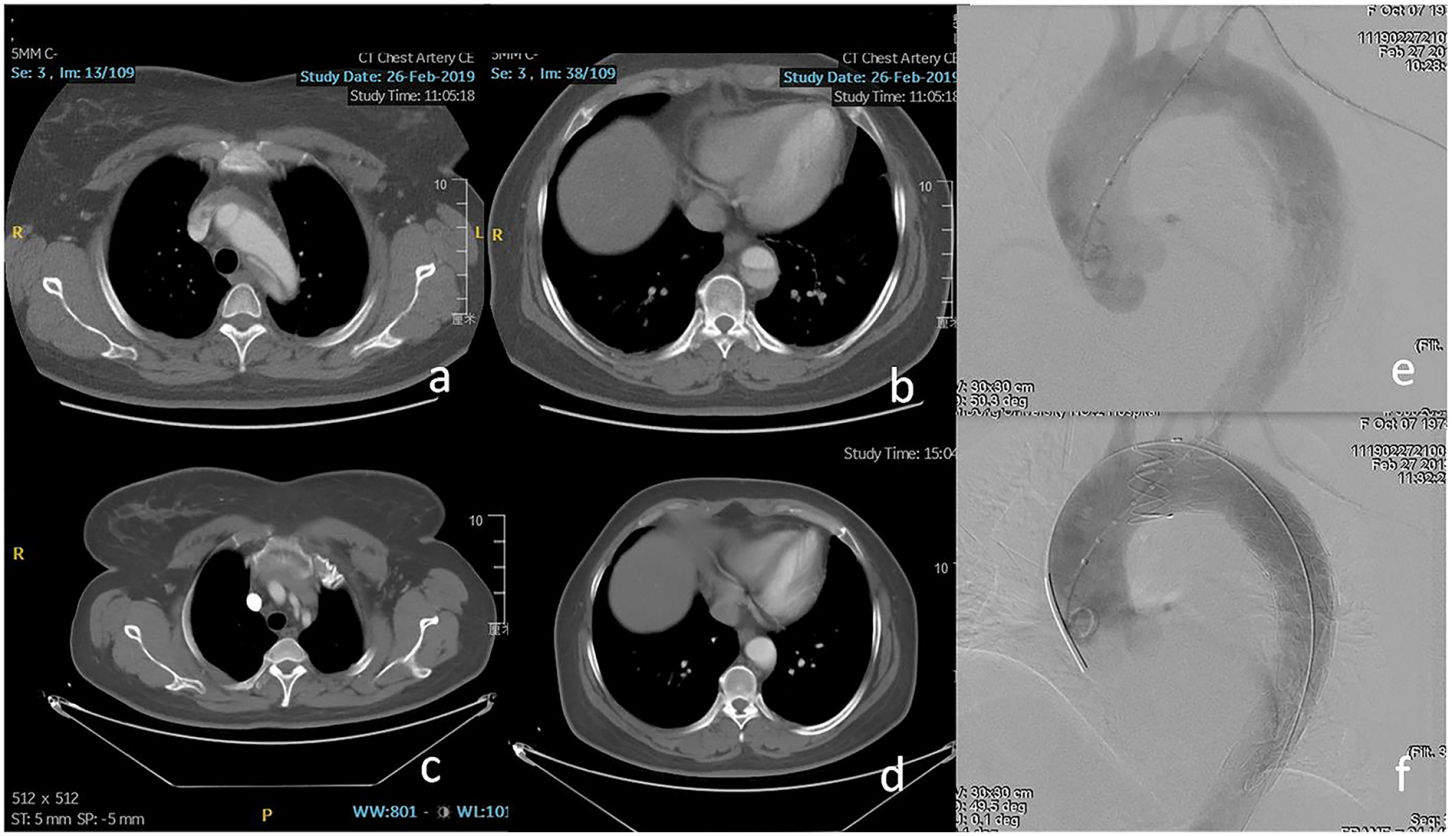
(a,b) Retrograde dissection involved the inner curve of zone 2 and the visceral segment of the aorta. (c,d) Eight months after TEVAR, the patency of the LSA and complete thrombosis of the segment distal to the stent-graft was found on computed tomography angiography (CTA). (e,f) The fenestrated stent-graft was deployed in zone 2 to exclude the entry tear and preserve the LSA.
